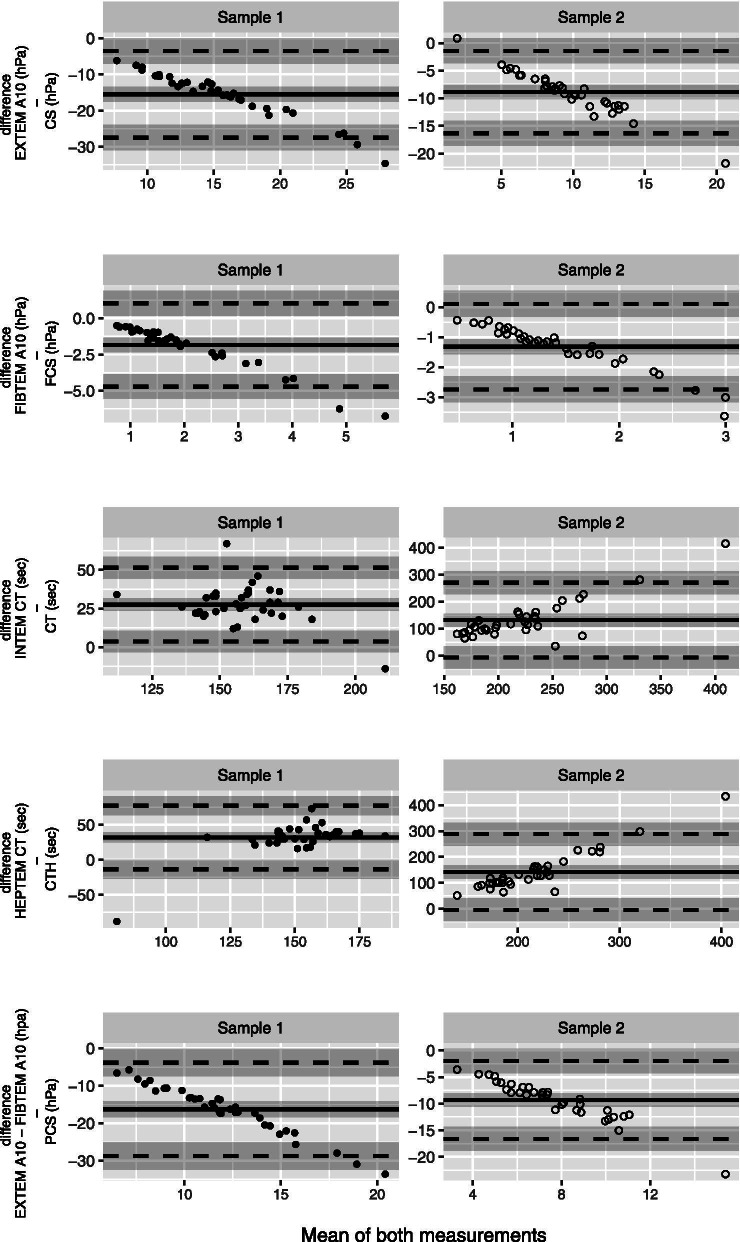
Bland Altman analysis. Plots for comparison of corresponding measurements of the Quantra and ROTEM sigma for sample 1 and 2 (EXTEM A10 and CS; FIBTEM A10 and FCS, INTEM CT and CT, HEPTEM CT and CTH; EXTEM A10-FIBTEM A10 and PCS). Data pairs of sample 1 are presented as solid circles and data pairs of sample 2 are presented as open circles. Y-axis represents the bias (difference of the methods) and X-axis the mean of both measurements. The mean bias (mean difference of the methods) is shown as solid line and the 95% limits of agreement is shown by the dashed lines. *Abbreviations:* CS, Clot Stiffness of the Quantra in hectopascal (hPa); FCS, Fibrinogen Contribution to Clot Stiffness of the Quantra (hPa); CT, Clot Time in seconds (sec) of the Quantra; CTH, Heparinase Clot Time (sec) of the Quantra; PCS, Platelet Contribution to Clot Stiffness (hPa) calculated by subtracting FCS from CS of the Quantra. EXTEM A10, clot stiffness of the ROTEM after 10 min running time (hPa); FIBTEM A10, fibrinogen contribution to clot stiffness of the ROTEM after 10 min running time (hPa); INTEM CT, Clot time of intrinsic pathway of the ROTEM (sec); HEPTEM CT, Clot time of the intrinsic pathway after neutralization of heparin of the ROTEM (sec); EXTEM A10 – FIBTEM A10, Platelet contribution to clot stiffness of the ROTEM after 10 min running time (hPa); Platelets, platelet count (10^9^/L)
